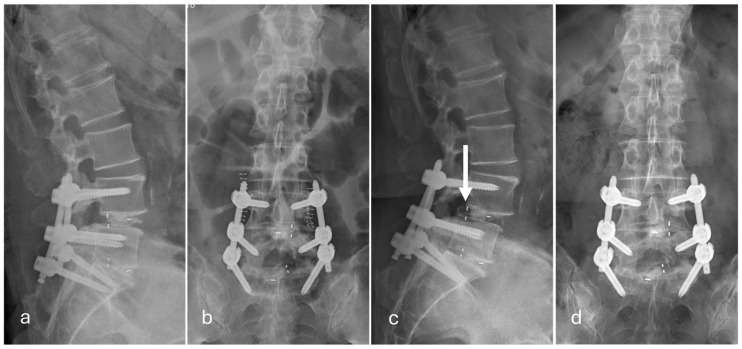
Representative radiographs following minimally invasive transforaminal lumbar interbody fusion at L4/L5 and L5/S1. (a,b) Postoperative radiographs obtained on postoperative day 3 demonstrate appropriate interbody cage positioning at both levels. (c,d) Follow-up radiographs at one month after surgery show posterior migration of the interbody cage at the L4/L5 level (arrow). No revision surgery was performed because the patient remained asymptomatic.
